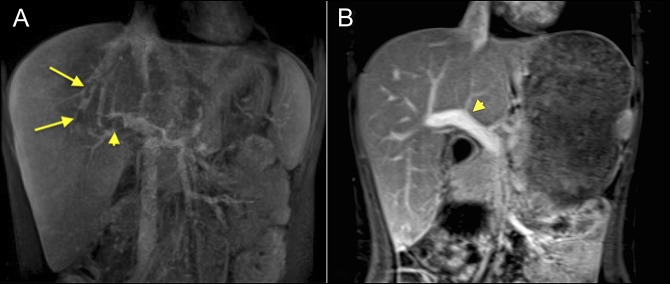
Coronal view of abdominal magnetic resonance imaging (MRI) (A) at admission showing filling defects within the right hepatic vein (arrows) and right portal vein (arrowhead) and (B) follow-up showing a near resolution of thrombus in the portal vein (arrowhead).
